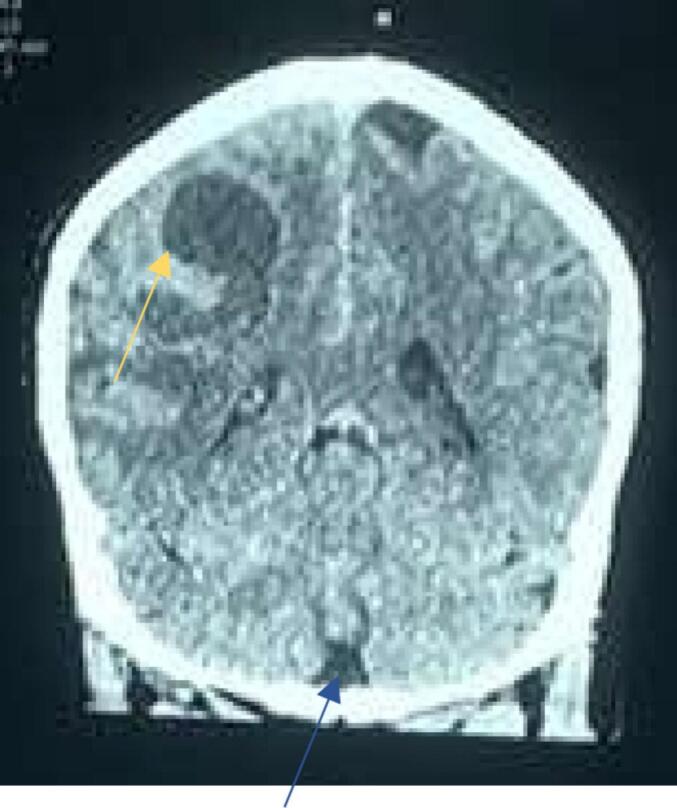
CT scan of brain showing Meningioma (blue arrow) and Glioma (yellow arrow). (For interpretation of the references to colour in this figure legend, the reader is referred to the web version of this article.)
